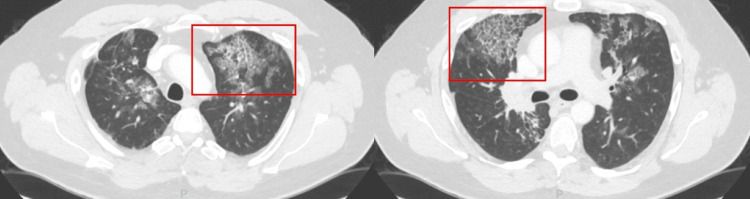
CT of the chest with intravenous (IV) contrast demonstrating bilateral areas of interlobular septal thickening.
